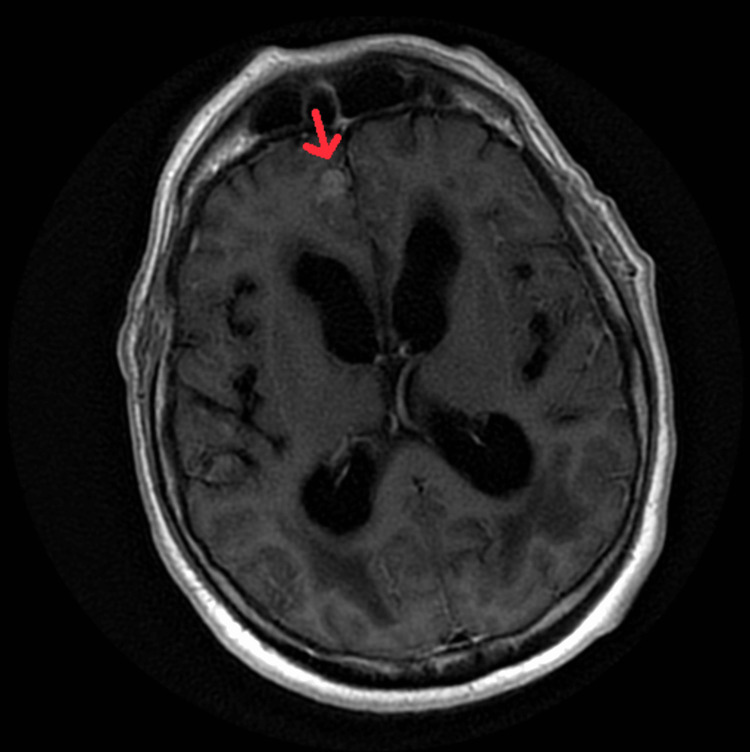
MRI of the brain with and without contrast, non-fat saturated post-contrast T1-weighted image, shows meningeal enhancement within the sulci of the right frontal lobe.
